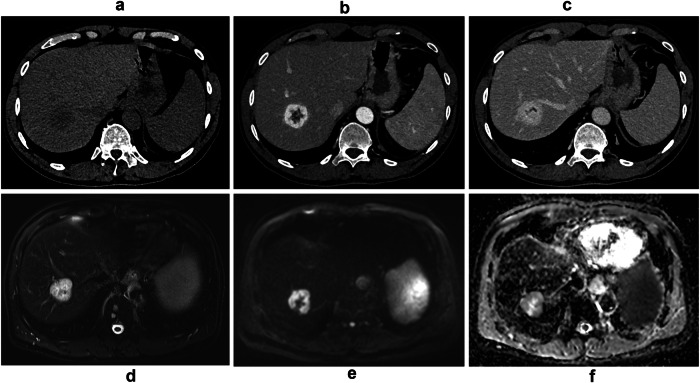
A 58-year-old male with an incidental liver lesion shows the typical LGVNL pattern. a–c CT scan shows (a) hypoattenuating lesion of segment VII, (b) thick peripheral lobulated arterial phase “flower petal shape” enhancement, (c) progressive centripetal portal phase filling. d, e On MRI, the lesion shows (d) marked T2 hyper-intensity on fat-suppressed T2-weighted image, (e) hyper-intensity on high-b value DWI (b = 900) with (f) no apparent diffusion coefficient restriction
